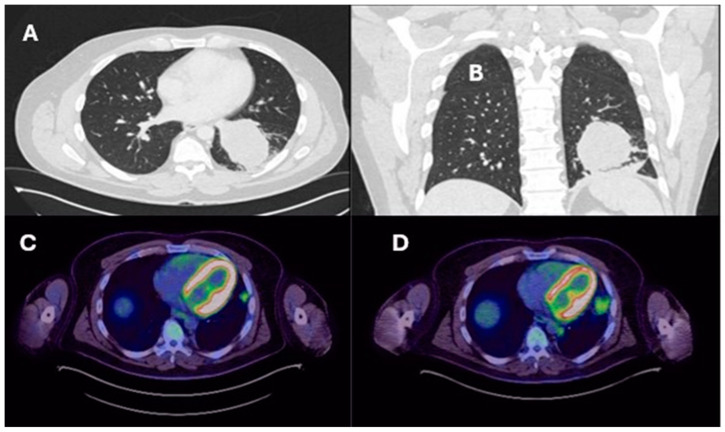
Contrast-enhanced chest CT, axial section demonstrating a large left lower lobe lung cancer (A), coronal view (B), post-resectional recurrence on PET-CT, axial section (C), and recurrence in the station 8 paraoesophageal region (D).
